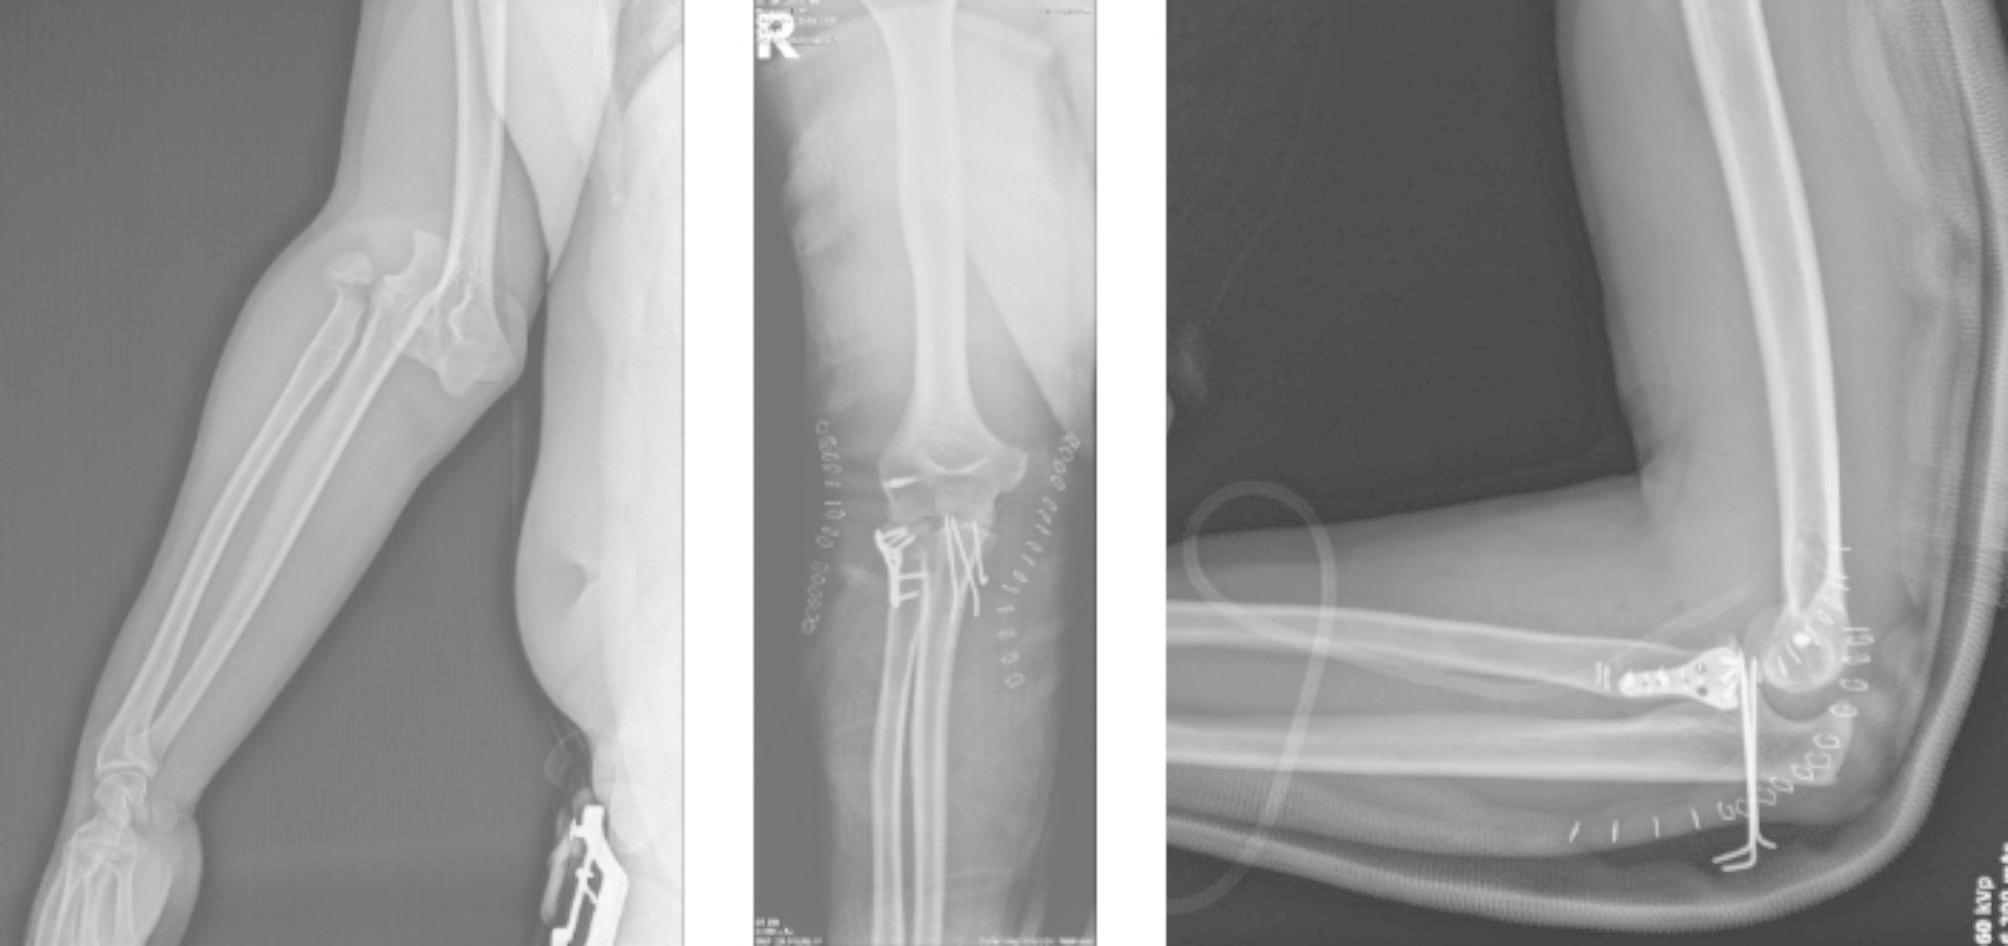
A 23-year-old male patient with elbow fracture dislocation (terrible triad) after falling from e-scooter. (A) Preoperative antero-posterior radiography, (B) Postoperative antero-posterior radiography, (C) Postoperative lateral radiography
